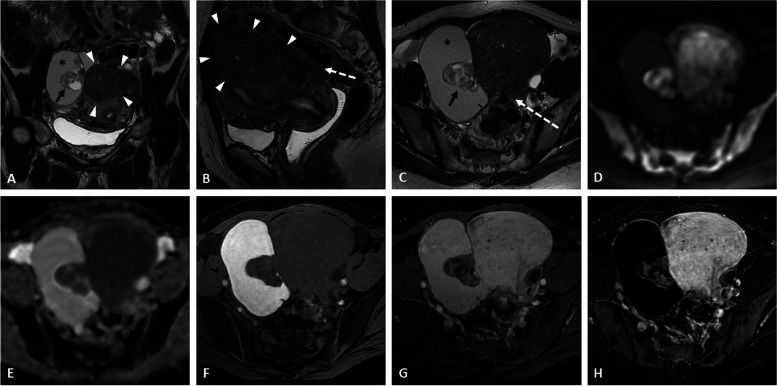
High-grade serous adenocarcinoma within an endometrioma in a 48-year-old woman with a known history of endometriosis. Coronal (a), sagittal (b), and axial (c) T2-weighted images show severe uterine adenomyosis (white arrowheads) and deep endometriosis involving the posterior compartment (dotted arrows). There is a mixed cystic right ovarian mass (asterisk) with intermediate signal intensity on T2 images (shading effect) containing a mural nodule (black arrows). Diffusion-weighted imaging (d high *b* value and e ADC map) shows a restricted diffusion within the mural nodule. Axial fat-suppressed T1-weighted image (f) shows the typical features of ovarian endometrioma. Heterogeneous enhancement of the nodule is displayed on fat-suppressed contrast-enhanced T1 image (g) and subtraction sequence (h)
